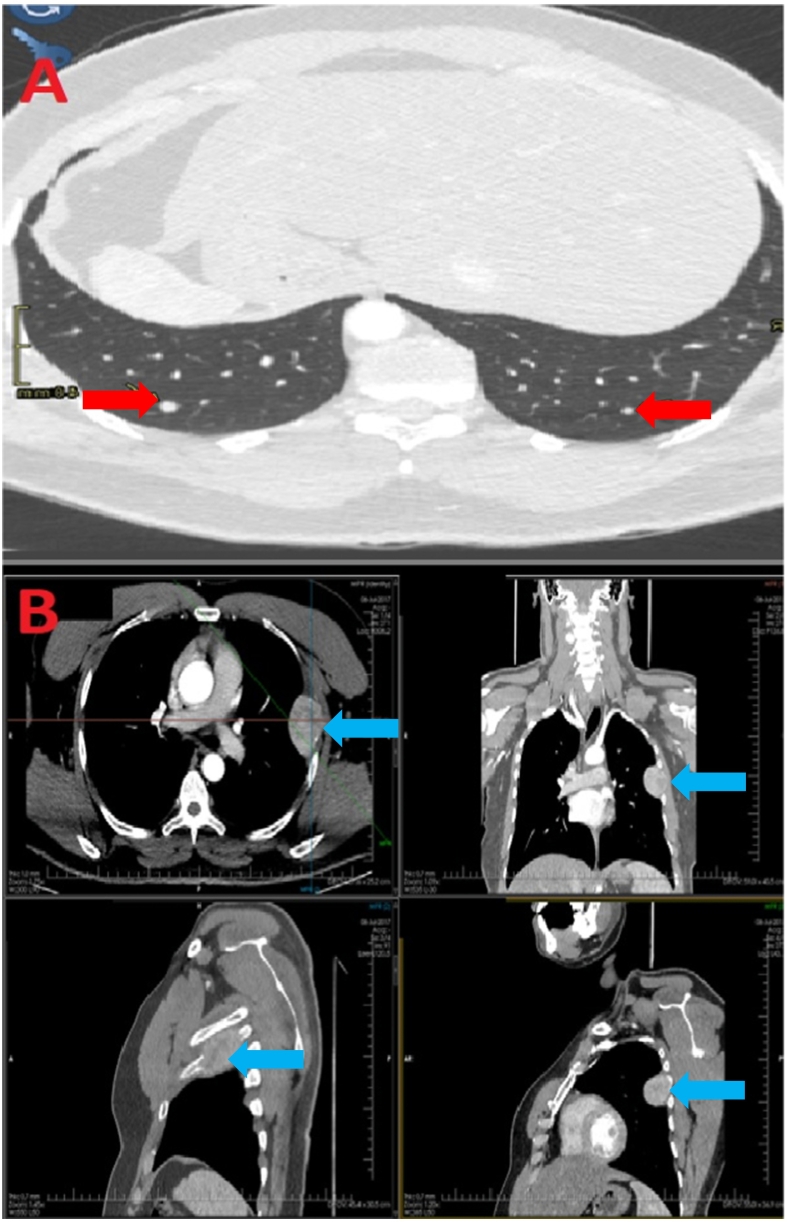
A - Metastatic work up axial view of CT chest lung window showing bilateral metastatic pulmonary nodules (red arrow). B – Metastatic work up reformatted CT chest images soft tissue window showing an enhancing lesion affecting the left 5th rib (blue arrow).
